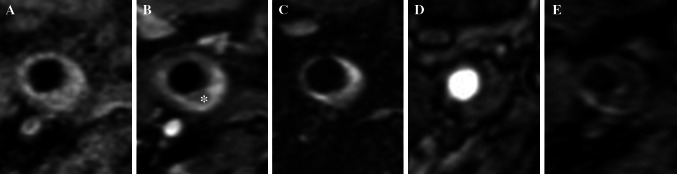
MRI allows depiction of several atherosclerotic components including lipid core (*asterisk*). Signal hypointensity (*asterisk*) indicates the lipid core of an eccentric atherosclerotic plaque with luminal preservation on fat-saturation T1-weighted imaging pre- (a), and post-contrast (b). Multi-contrast images usually also include T2 fat saturation (c), time-of-flight (d), and MPRAGE (e)
